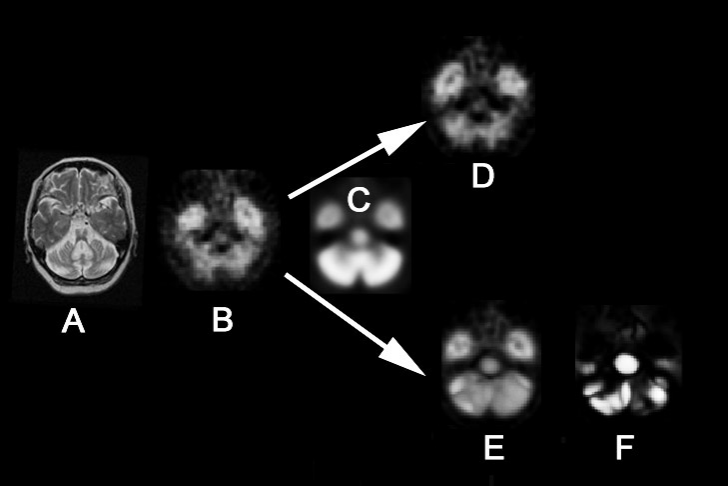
Conventional warping and high-dimension-warping of a SPECT image in an MSA-C patient. T2-weighted MRI (A) showed severe atrophy in cerebellum and pons in a 56-year-old woman with MSA-C. A SPECT image at the level of the pons (B) demonstrated perfusion decrease in the cerebellum and pons. In contrast to conventional warping (D), high-dimension-warping (E) achieved sufficient spatial normalization to a template (C) using a contraction map (F).
